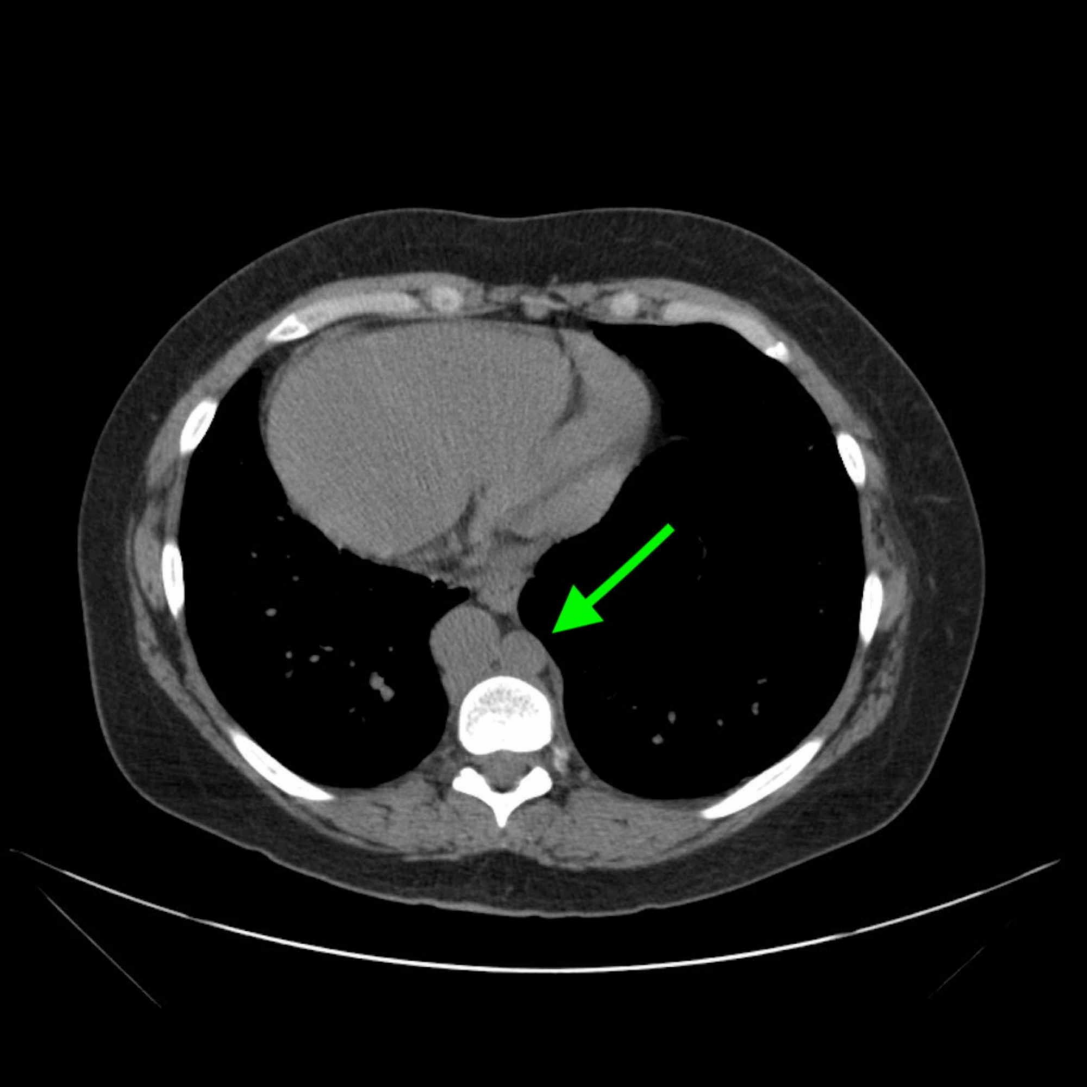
Inferior vena cava with azygos vein continuation on abdominal CT without contrast.
